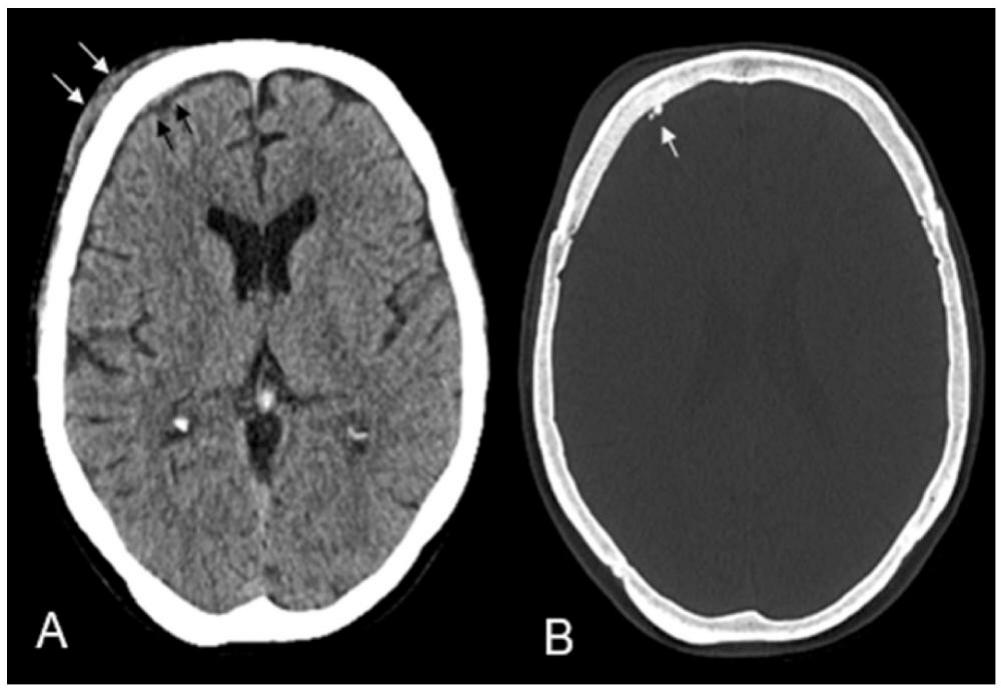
The preoperative CT scan revealed the extracranial subgaleal soft tissue component of the tumour (arrows A) and tiny calcifications along the thickened and enhancing right frontal dura (arrow B). However, the bone marrow of the frontal skull appeared unaffected.
